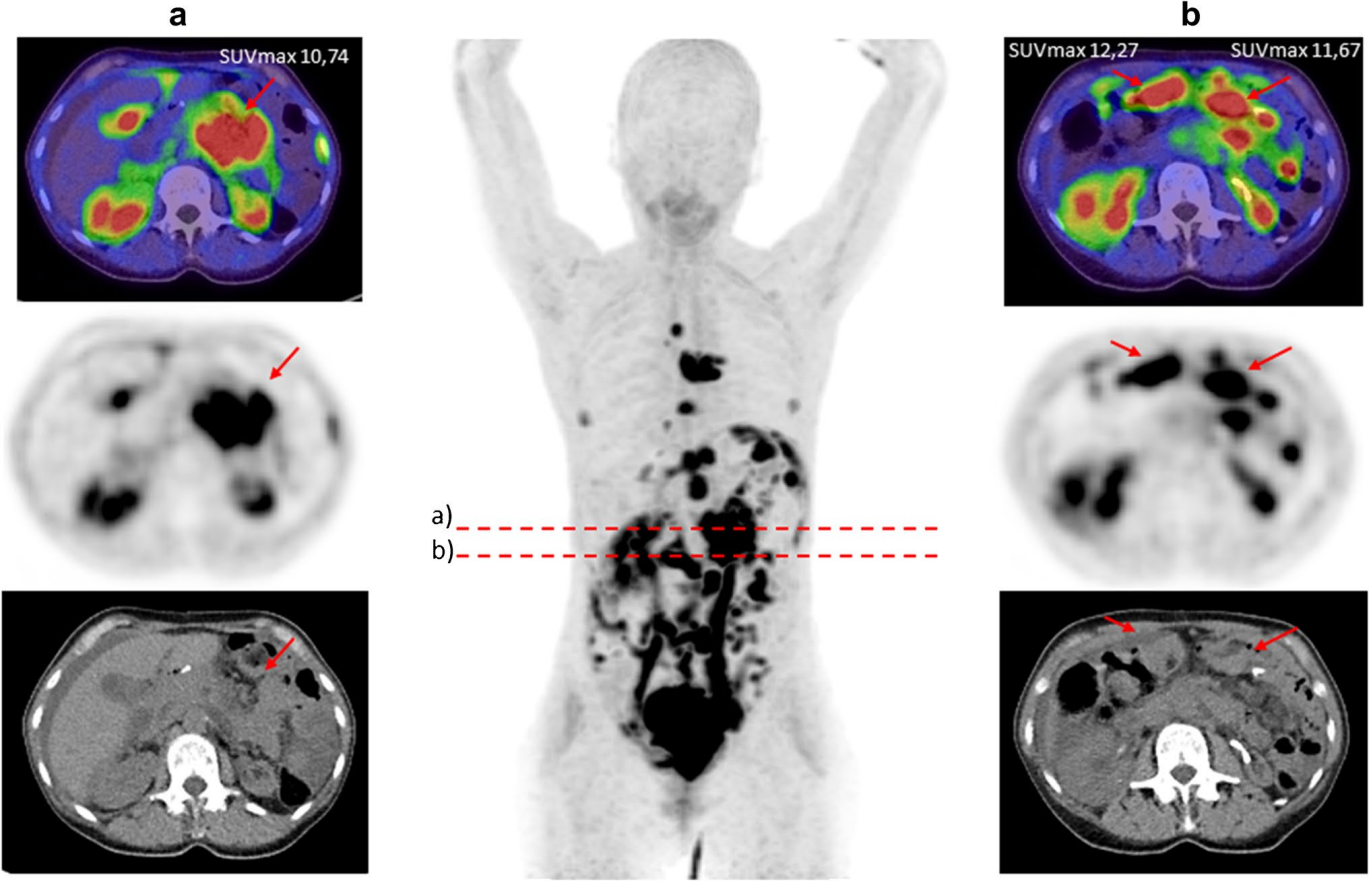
A 60-year-old female patient with gastric cancer and peritoneal carcinomatosis and lymph node metastases underwent ^68^ Ga-FAPI-PET/CT due to staging after gastrectomy. Tracer uptake of the peritoneal carcinomatosis presented an excellent median SUVmax of 11.91
